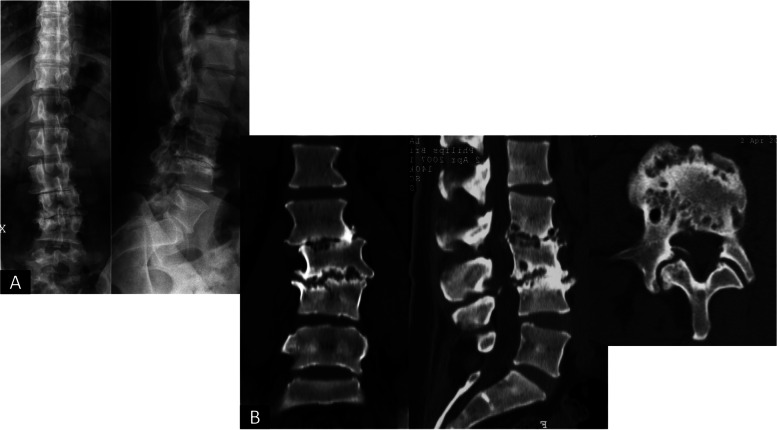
A and B: Evolution of the deformity in the x-rays and increased endplate erosion (A), which involved L2 as well as shown in the CT scan (B-April 2007)
